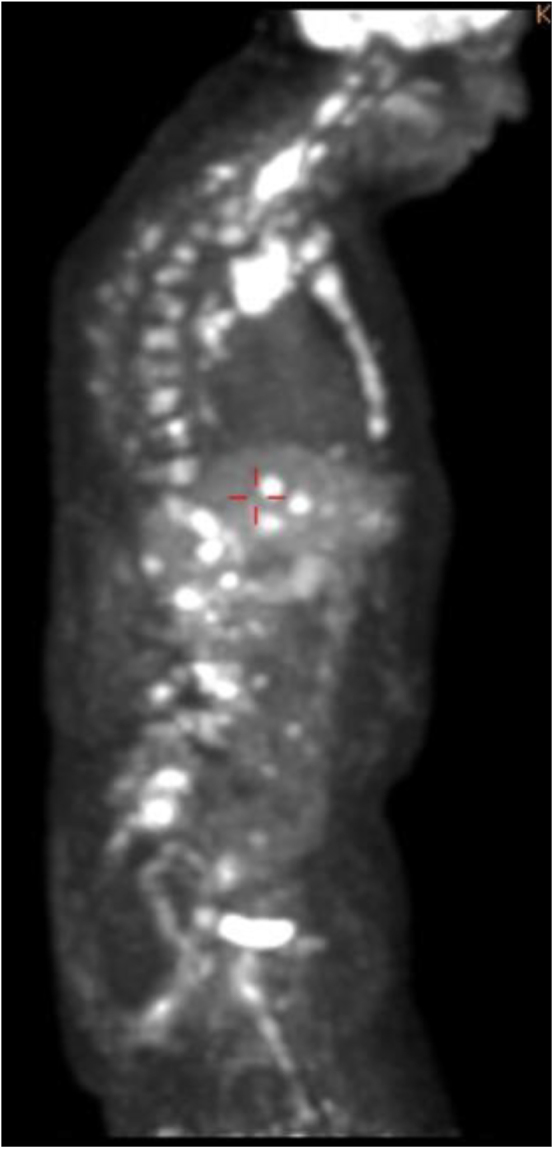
Fludeoxyglucose-18 (FDG) PET scan showed extensive multisystem metastatic
